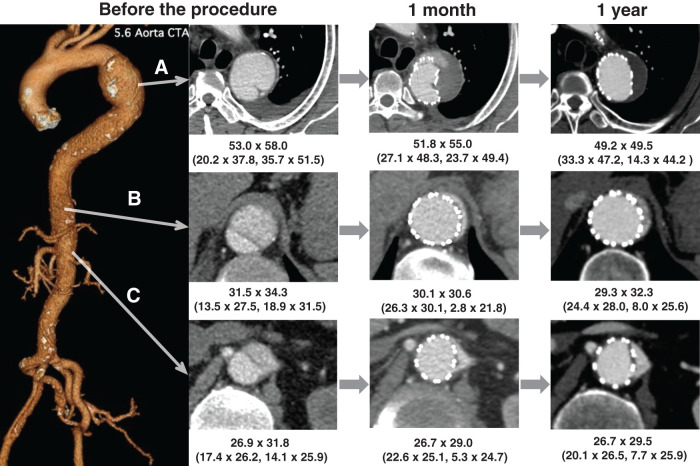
CT images of case 3 at 3 time points: preoperatively, 1 month, and 1 year after the STABILISE procedure. Aortic diameter (short axis × long axis, mm); TL and FL diameters are shown in parentheses. (A) Thoracic descending aortic aneurysm at T4–T5 level. Preoperatively, a chronic dissection with the primary entry tear located at the distal aortic arch and a 58-mm aneurysm was observed. At 1 month postoperatively, type 1A endoleak was still present. At 1 year, the endoleak had disappeared and aneurysm sac shrinkage was observed. (B) Descending thoracic aorta just above the celiac artery. Preoperatively, FL was patent and TL was compressed. At 1 month postoperatively, TL was dilated, but a small patent FL remained. At 1 year, FL is still patent, but decreased in size. (C) Abdominal aorta at the origin of the renal arteries. Preoperatively, the left renal artery branched from both TL and FL. At 1 month postoperatively, TL was dilated and perfusion to the left renal artery was preserved, although a small patent FL remained. At 1 year, FL was still present without evidence of enlargement, and TL had further expanded. FL: false lumen; STABILISE: stent-assisted balloon-induced intimal disruption and relamination in aortic dissection repair; TL: true lumen; CT: computed tomography
